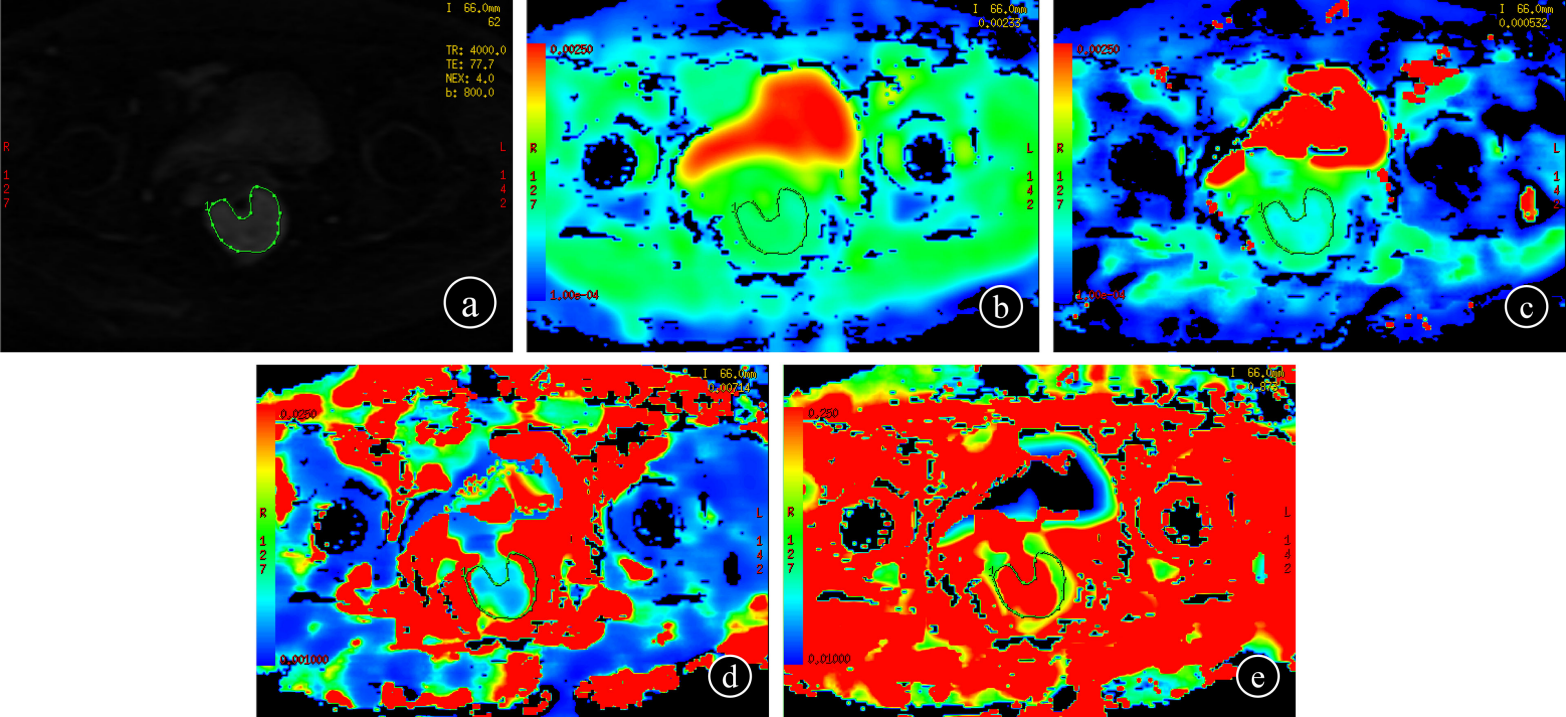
IVIM-DWI measurement (b = 800 s/mm^2^) (A); each radiologist drew ROI three times to get the values on the maps of ADC, D, D^∗^, and f, respectively (B–E).
